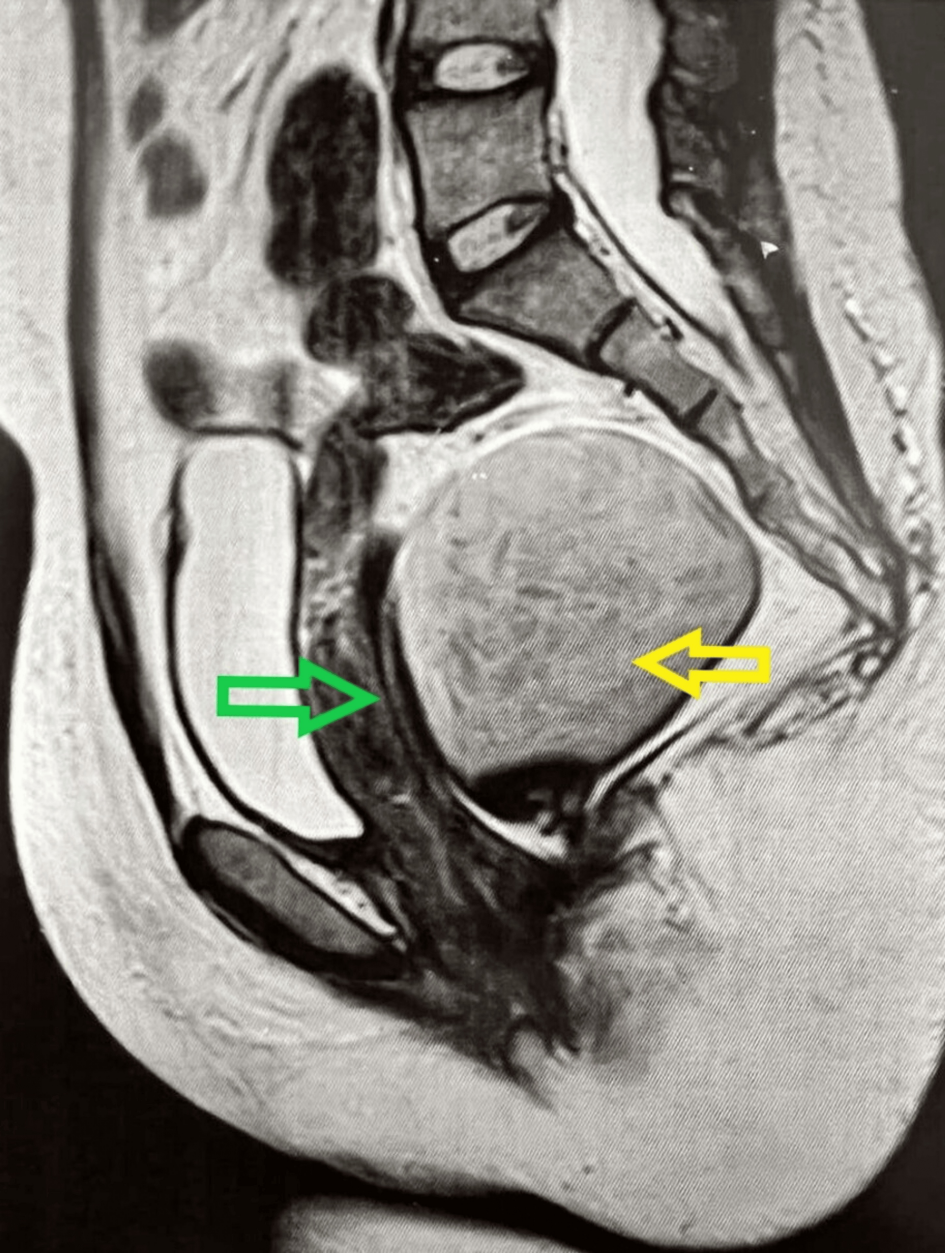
MRI of the pelvis showing tailgut cyst (yellow arrow) in the presacral space behind the rectum (green arrow)
